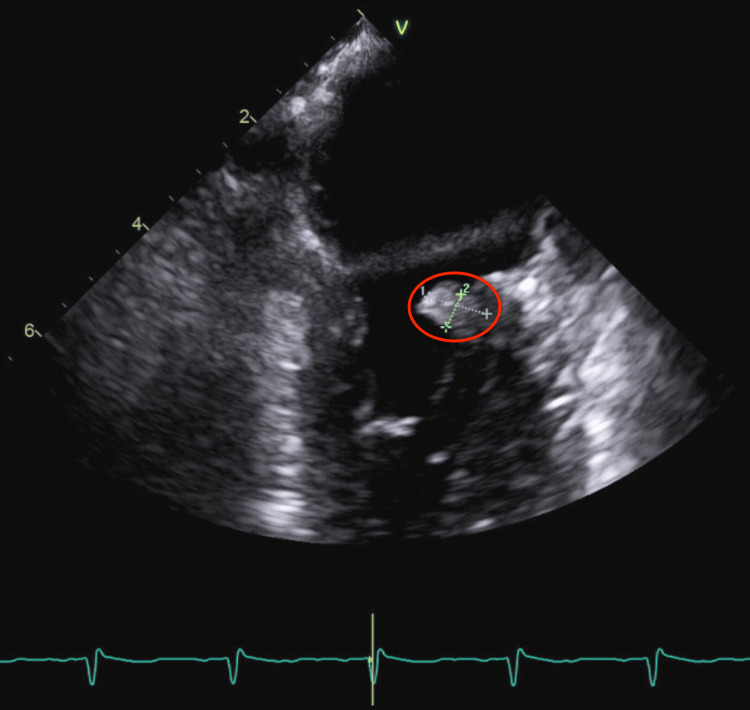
TEE, revealing a mitral valve vegetation TEE, transesophageal echocardiography
